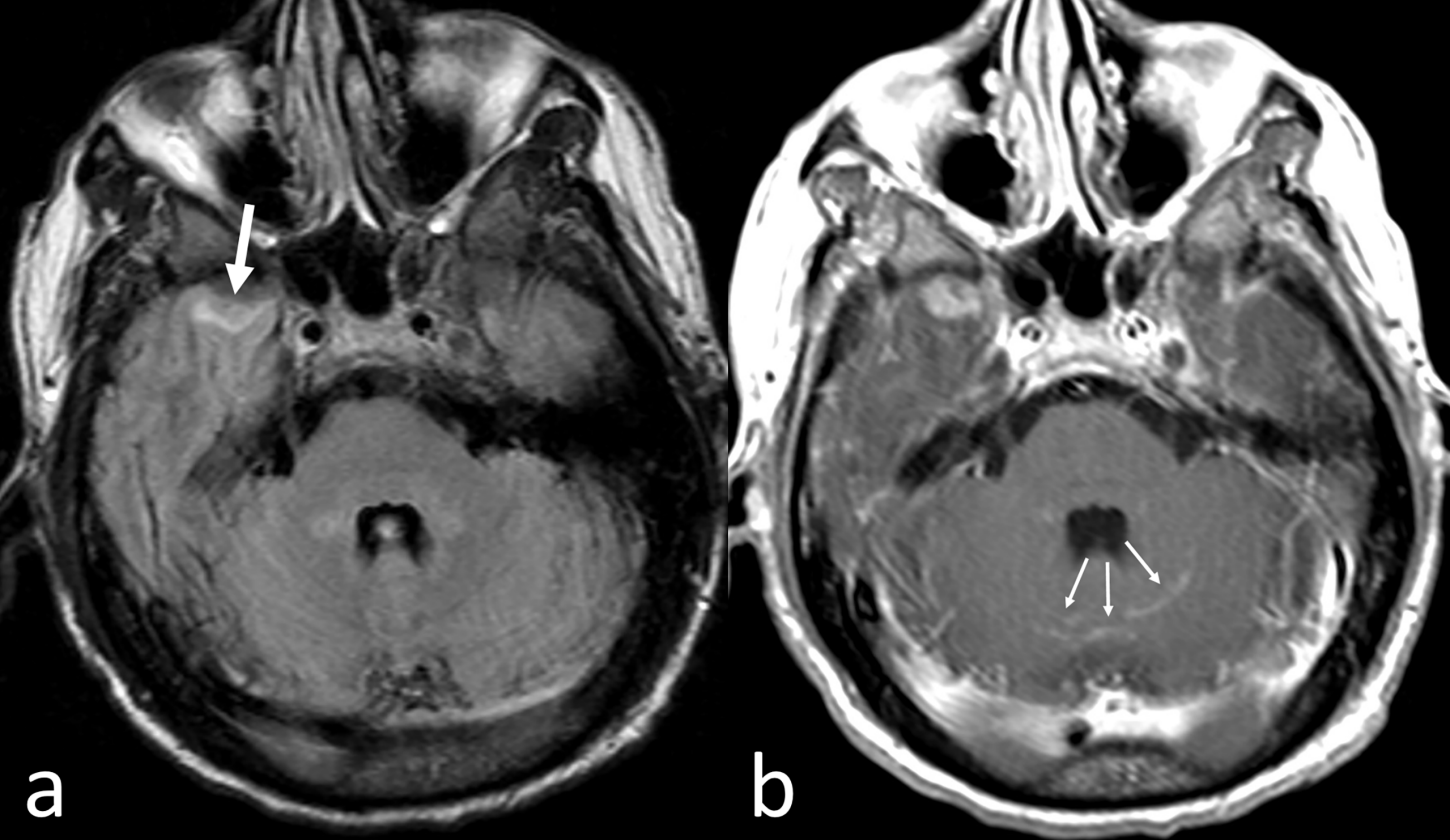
CNS-MM. Despite artifactual images from patient’s movement, fast fluid-attenuated inversion recovery axial MR image (A) shows a small area of abnormal intensity in the surface of the anterior pole of the right temporal lobe (thick arrow). Corresponding Gadolinium-enhanced T1-weighted axial MR image (B) shows enhancement not only of the left temporal lesion but also in some cerebellar sulci (thin arrows).
